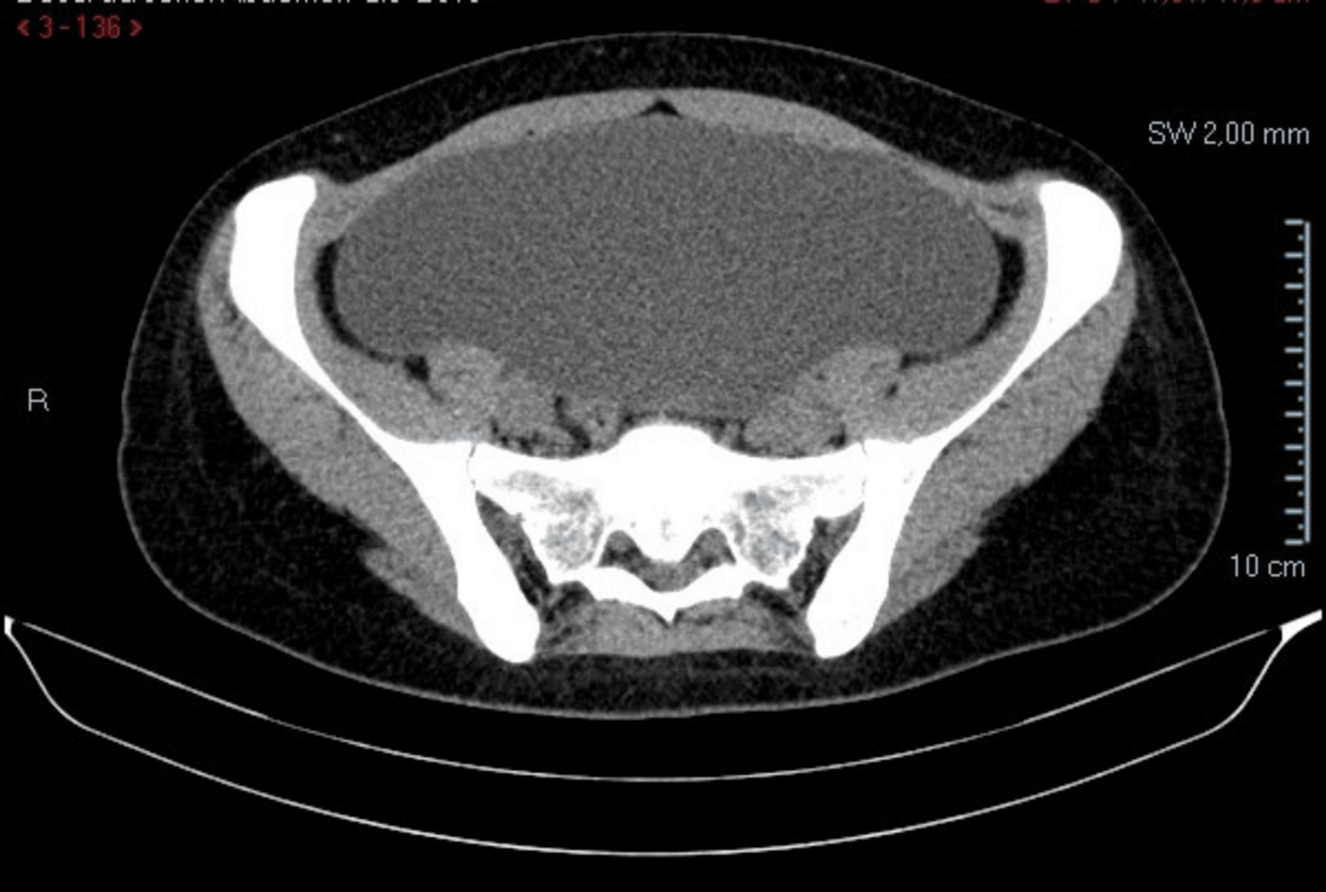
Abdominopelvic CT scan (axial section) of the cyst CT: computed tomography
